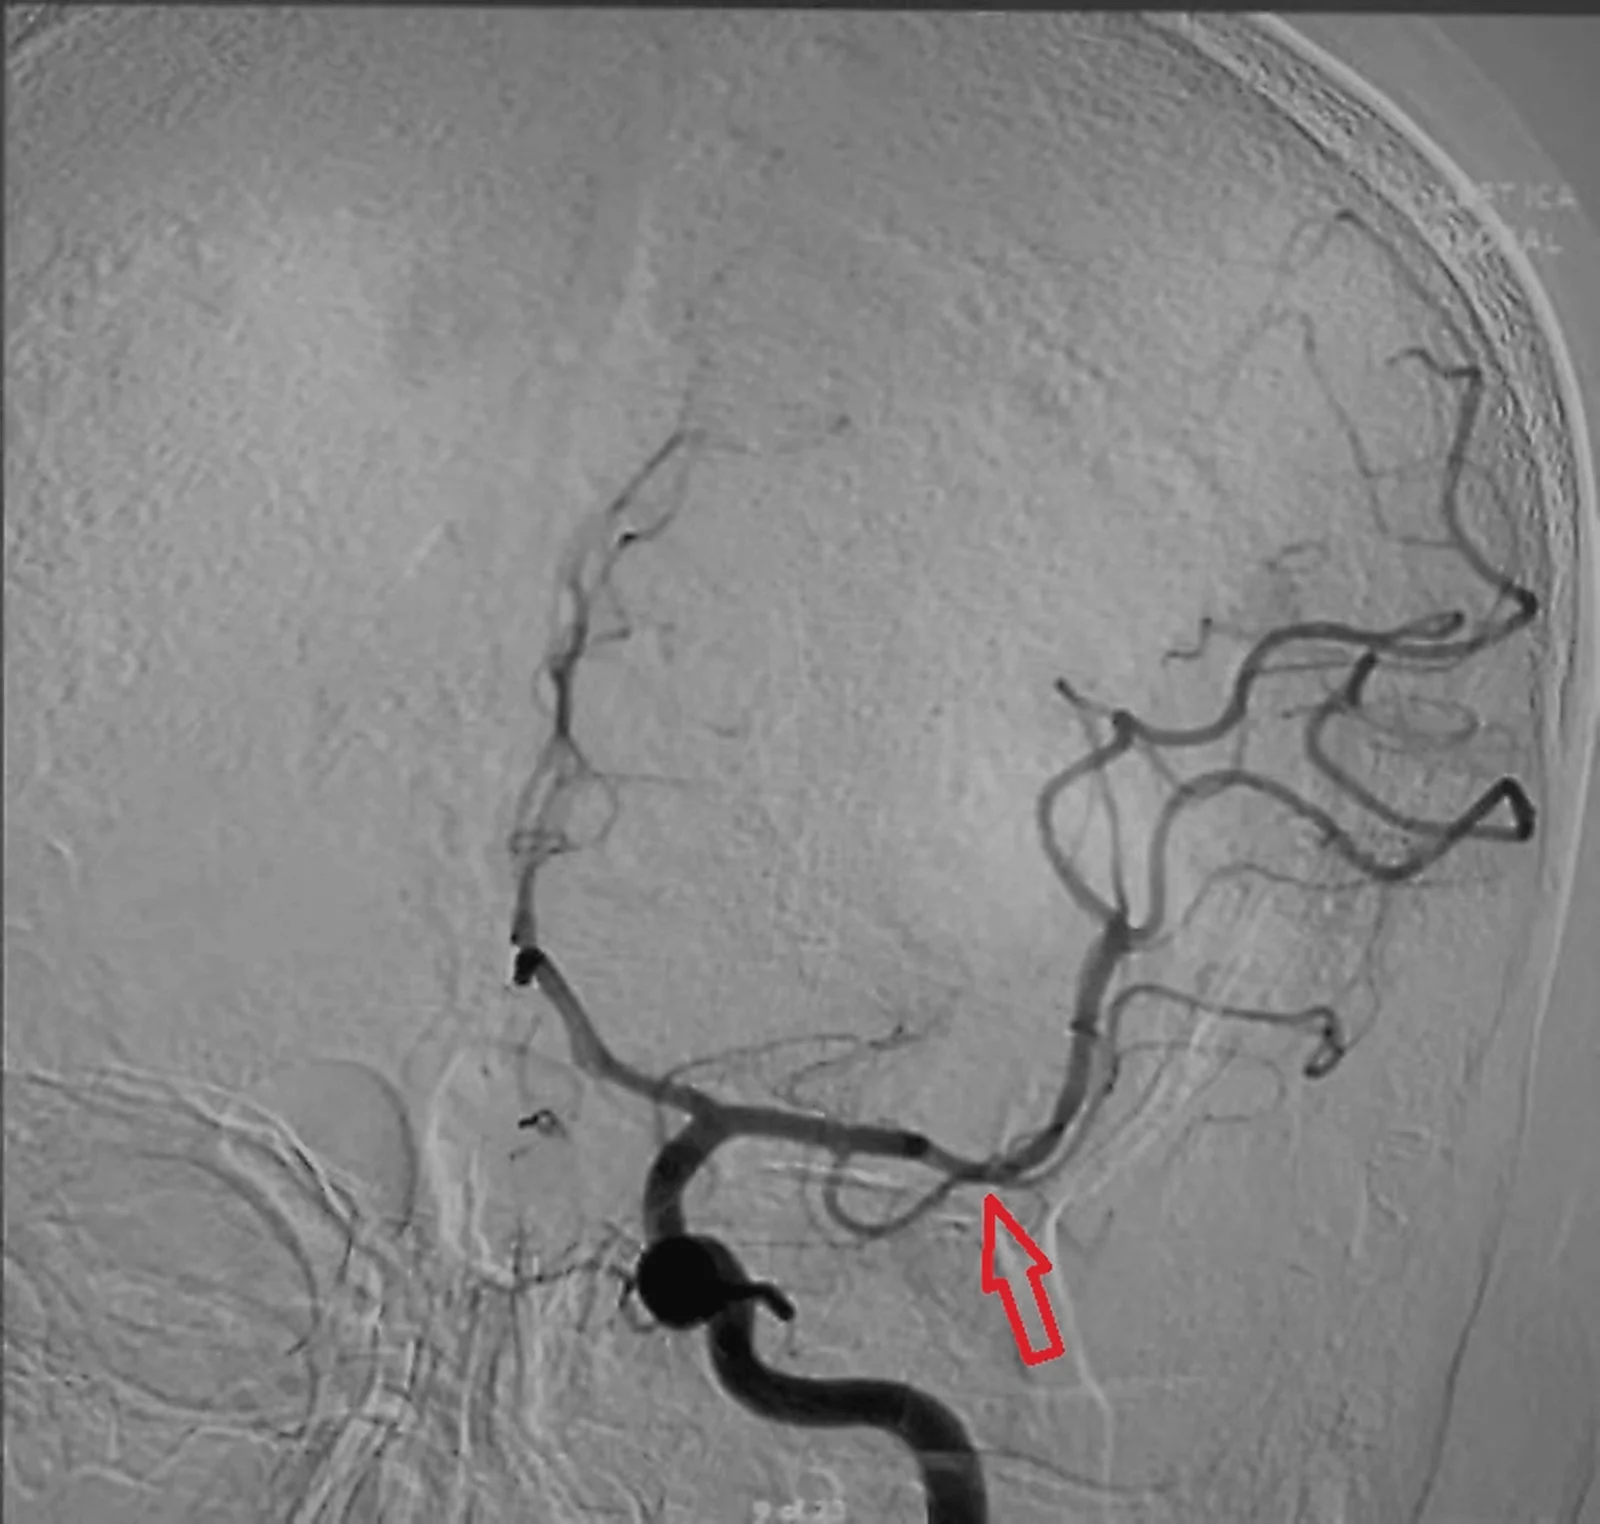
CT cerebral angiogram Initial cerebral angiography demonstrating left M2 middle cerebral artery occlusion (arrowed) prior to mechanical thrombectomy.
